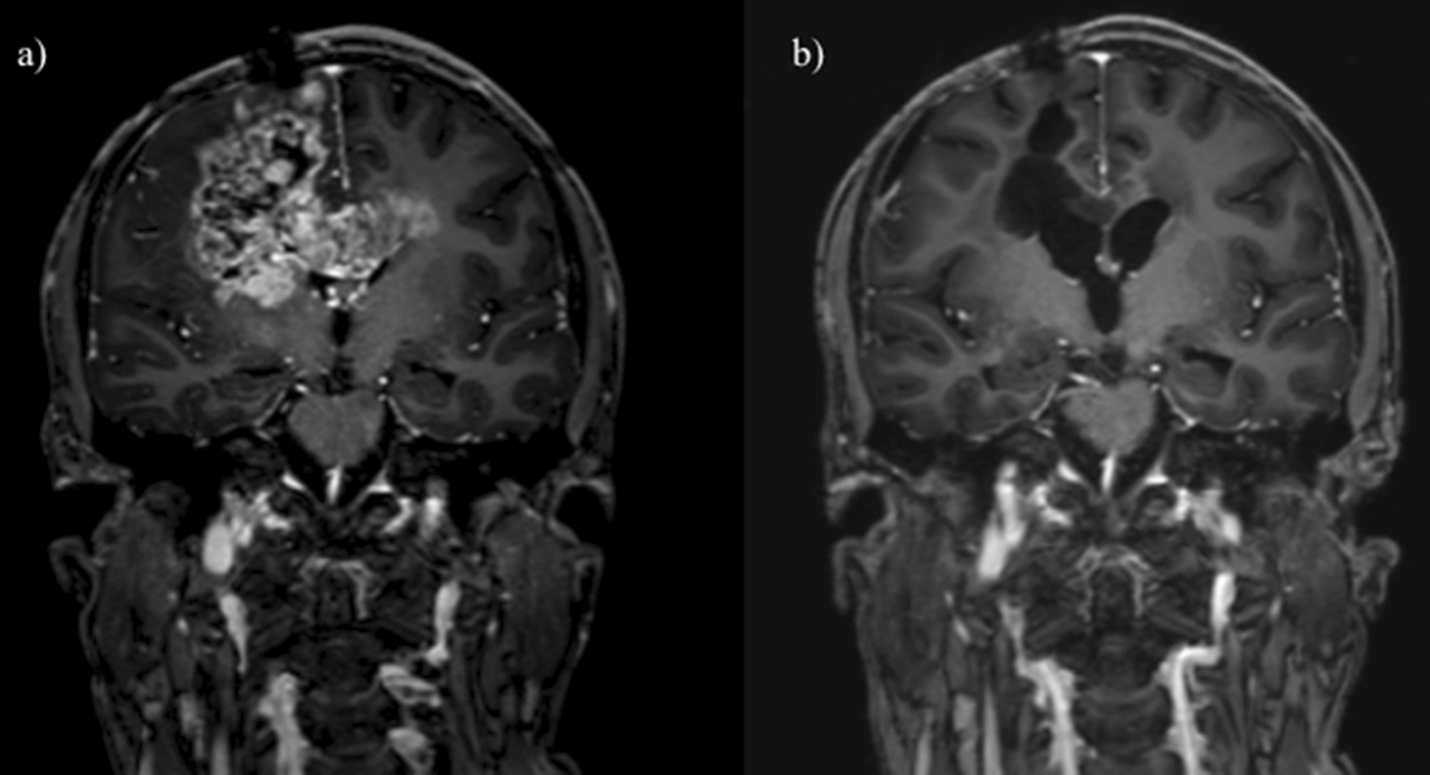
Patient 5: Contrast enhanced T1 weighted MRI, coronal plane, a) before, b) four weeks after reirradiation: partial remission of the relapsed tumor four weeks after a second course of radiation therapy with 17 × 1.8 Gy = 30.6 Gy.
